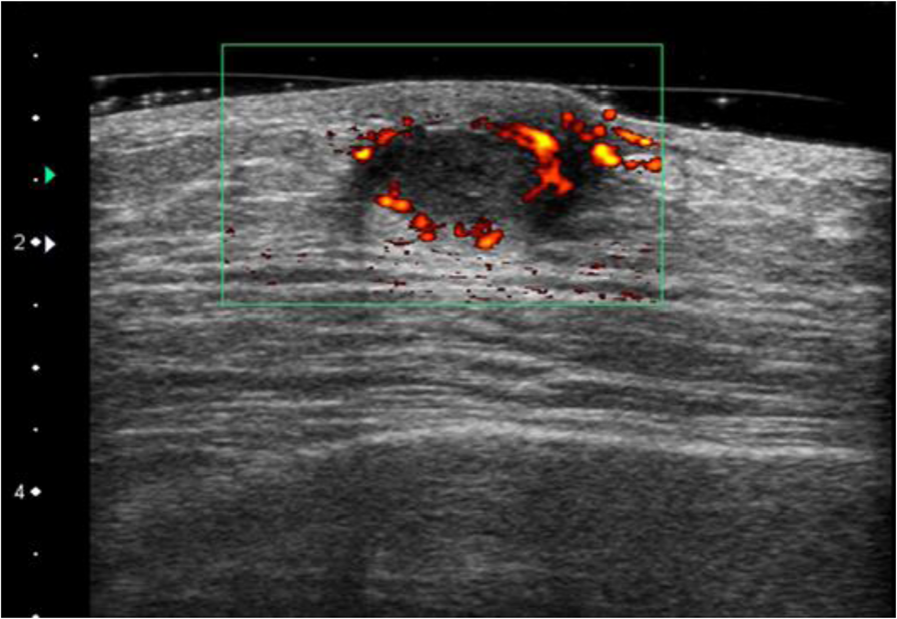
Ultrasonography revealed a hypoechoic lesion 16 mm in size, with accompanying posterior echo attenuation and blood flow. These findings were highly suggestive of a malignant tumor
